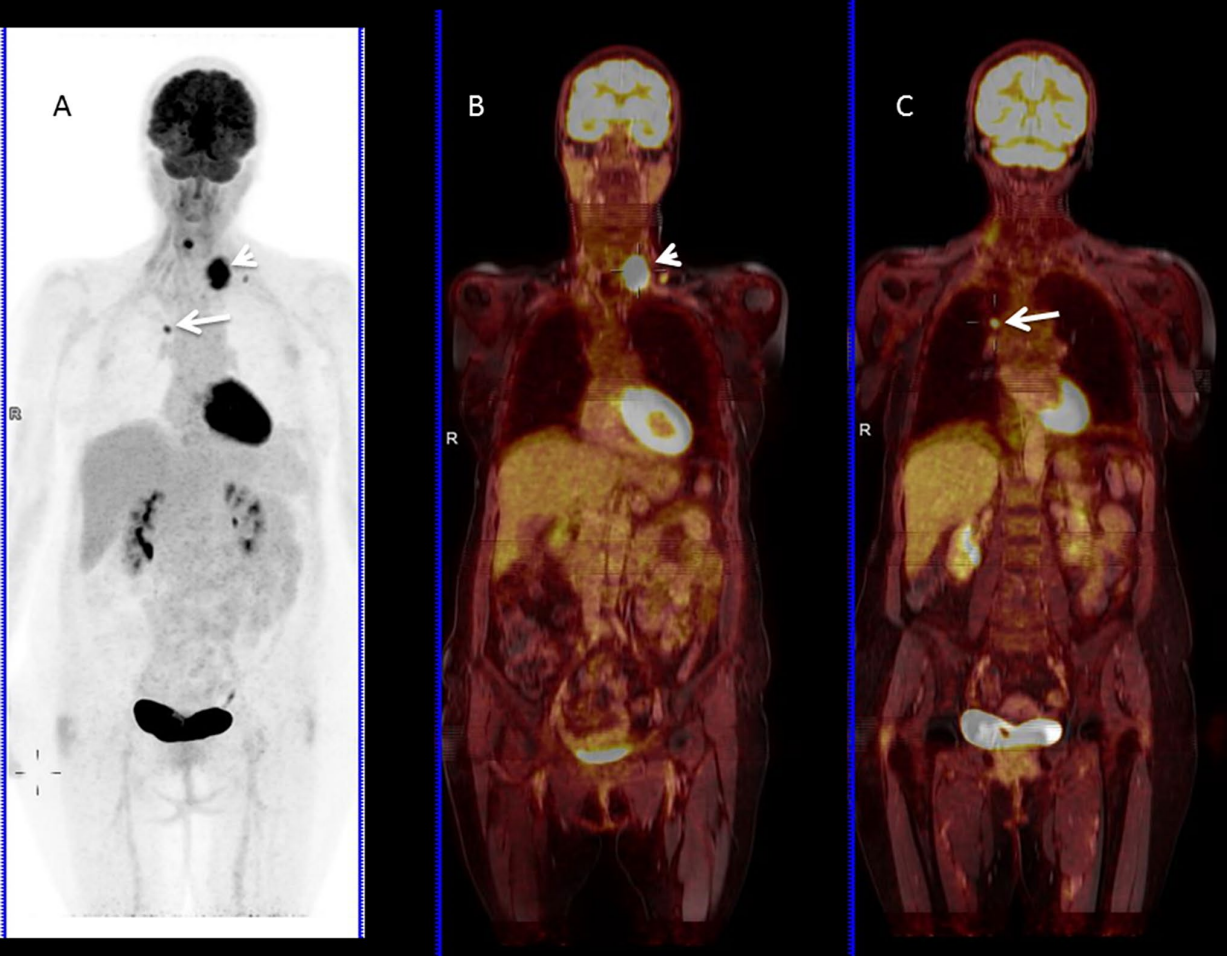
Follow-up of treated NSCLC with PET-MRI. a
